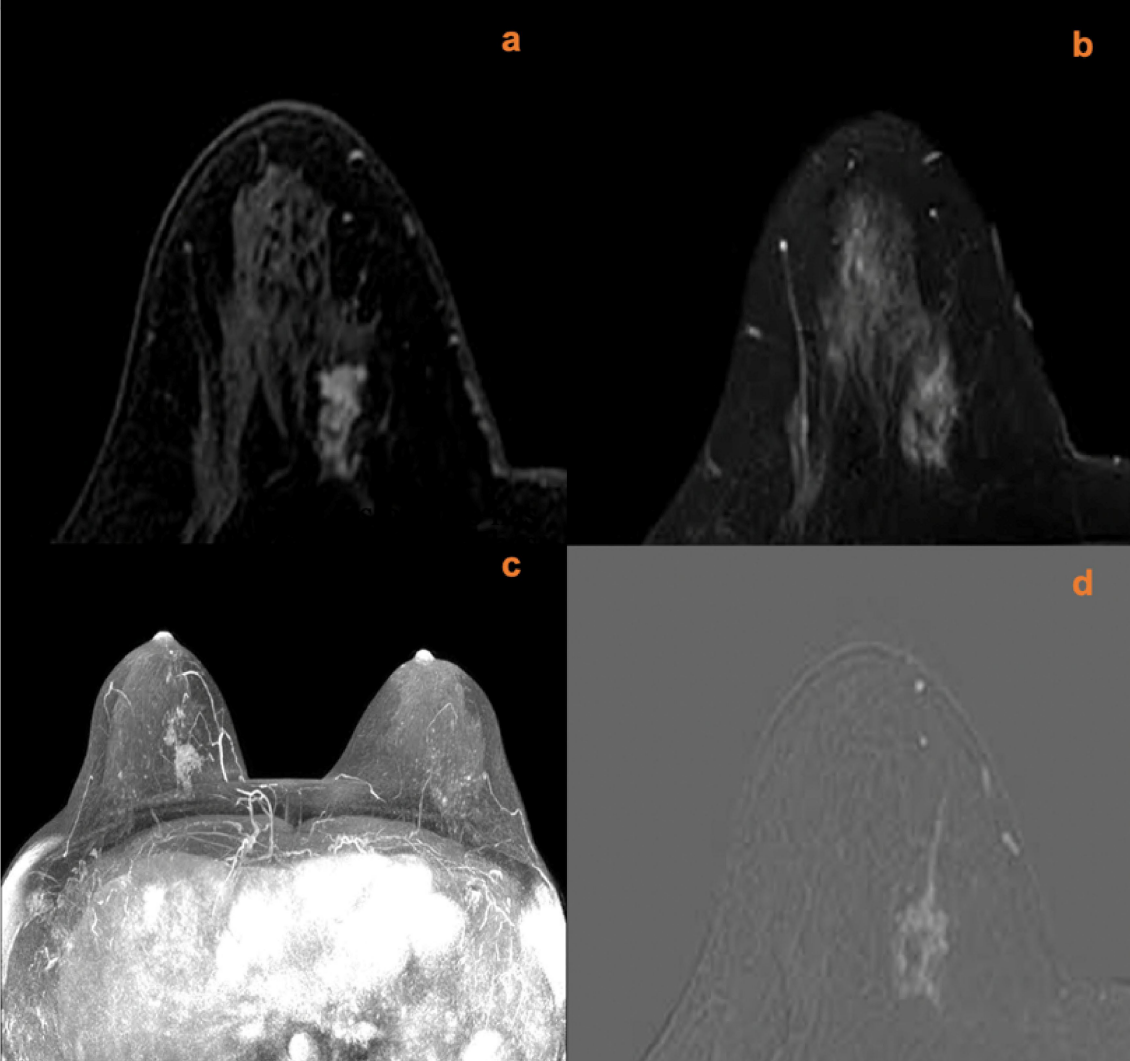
A 40-year-old female with right nipple discharge for two weeks. MRI (A, contrast-enhanced axial; B, T2w-SPAIR image; C, maximum intensity projections image; D, subtraction image) shows a right breast non-mass lesion with a root sign. There was no peripheral edema around the lesion. The lesion increased the ipsilateral breast vascularity and the positive AVS sign. The KS of the lesions was 7, while the KS^*^ was 10. Postoperative pathology confirmed that the lesion was an invasive ductal carcinoma.
